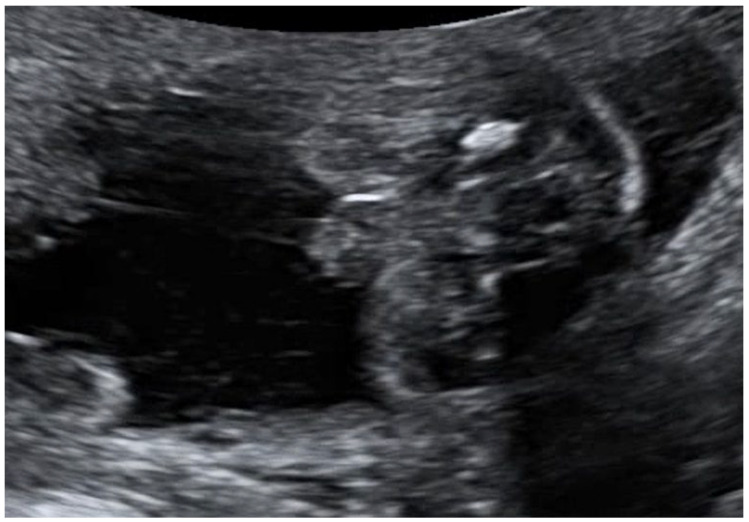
Ultrasound image of ambiguous genitalia characterized by the ‘tulip sign’, observed in a 46, XY fetus during the 20th week of gestation.
